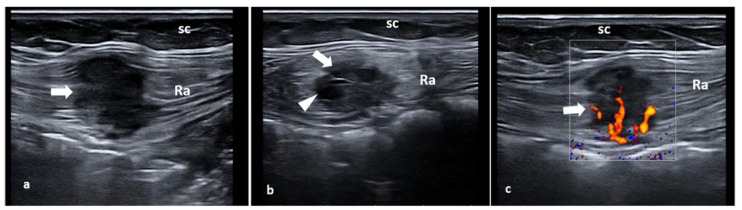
Long-axis sonograms show a hypoechoic, nodular mass inside the rectus abdominis with speculated borders (a). Short-axis sonograms show a partially cystic nodular (hypo-anechoic) mass with ill-defined margins (b). Fine intralesional vascular spots are depicted with color-power Doppler (c). sc: subcutaneous tissue ra: rectus abdominis muscle.
